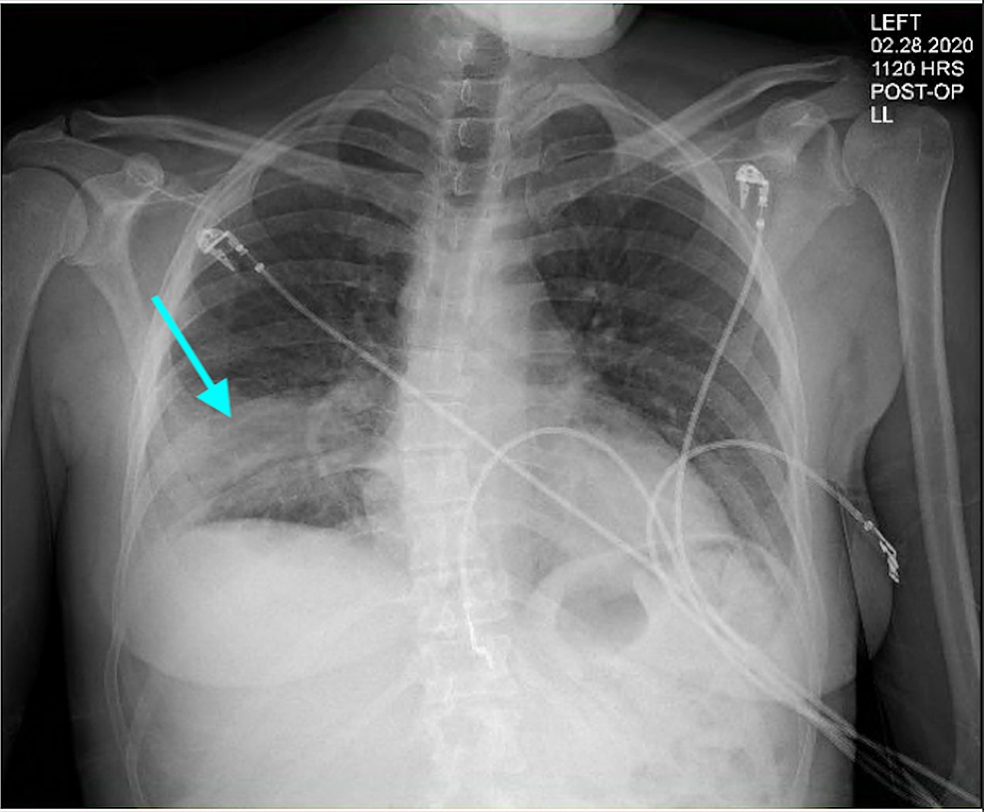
Chest X-ray showing right lower lobe lobar consolidation (blue arrow)
